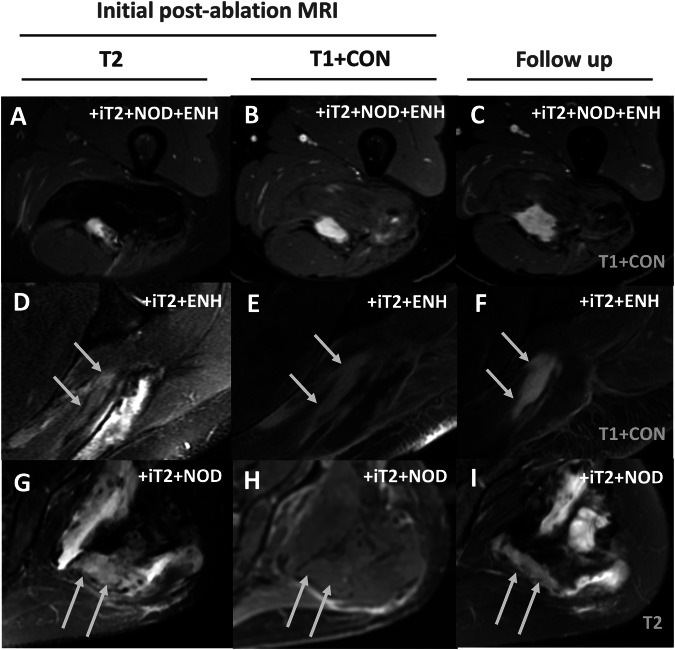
Examples of growing, unchanged, and regressing lesions in the context of the three subgroups with intermediate T2 signal. Column 1 is baseline T2 weighted FSE with fat saturation images (T2), while Column 2 is baseline T1 weighted SPGR fat-suppressed post-contrast images (T1 + CON). Column 3 is images from the last follow-up labeled as T2 or T1 + CON. (A–C: Top Row) 18-year-old female with a left posterior thigh desmoid tumor. Initial post-ablation imaging shows a nodule with intermediate T2 signal and enhancement. The nodule demonstrated growth on follow-up 365 days later. The patient underwent one HIFU and two cryoablations. (D–F: Middle Row) 59-year-old male with a left posterior shoulder desmoid tumor. Initial post-ablation MRI shows a non-nodular lesion with intermediate T2 signal and enhancement. The lesion was unchanged at follow-up 280 days following cryoablation. (G–I: Bottom Row) 55-year-old female with a left buttock desmoid tumor. Initial post-ablation MRI shows a non-enhancing nodule with an intermediate T2 signal. The nodule showed regression at follow-up 242 days later. The patient underwent two HIFU and one cryoablation. HIFU, high-intensity focused ultrasound under MRI guidance
